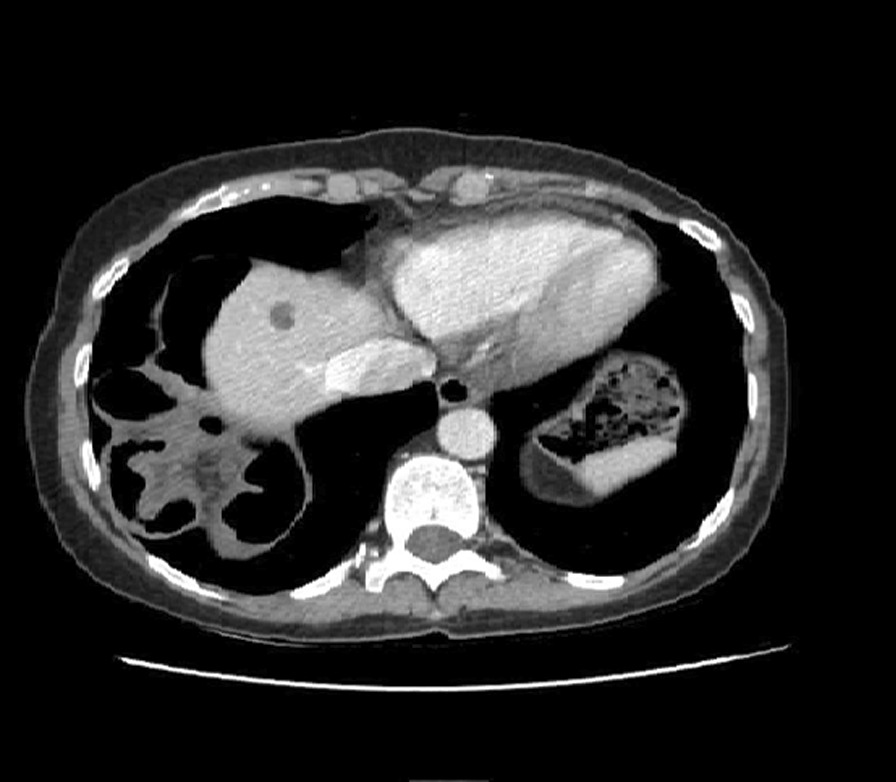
CT scan, right-sided diaphragmatic hernia
